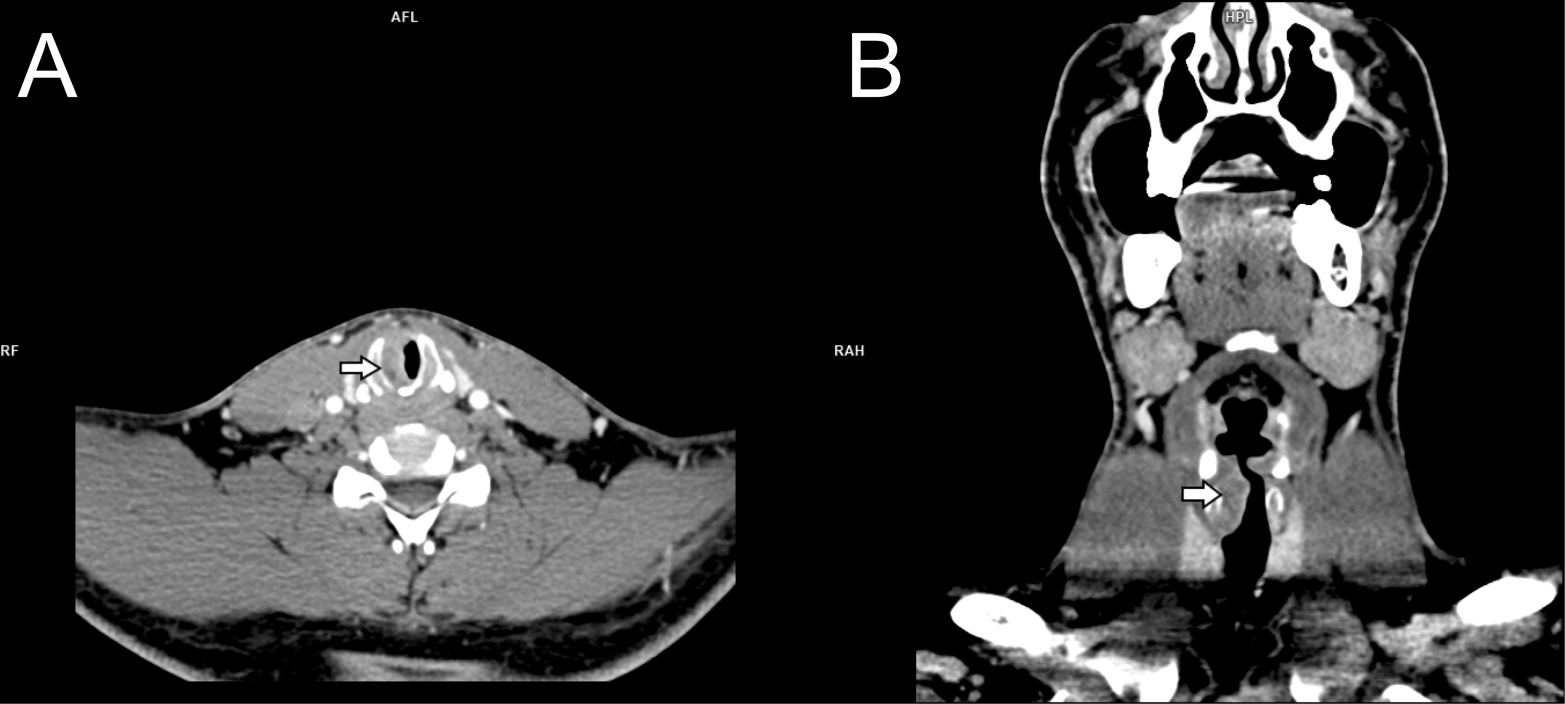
(A) Axial contrast-enhanced computed tomography shows right subglottic soft tissue mass (arrow) invading airway; (B) Coronal reformatted contrast-enhanced computed tomography shows mass (arrow) extension from right true vocal cord of glottis to the subglottic region.
